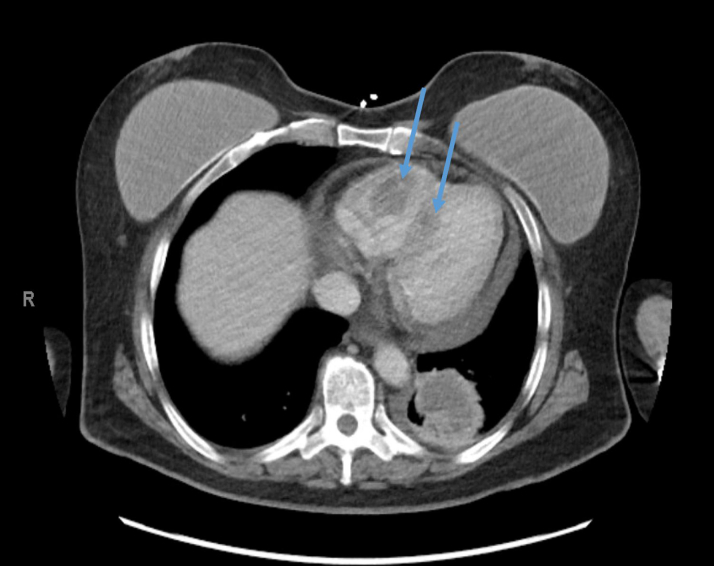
Computed Tomography of the Chest at Day 20 Showing Multiple Hyperintense Intracardiac Masses The blue arrows point to the intracardiac masses.
